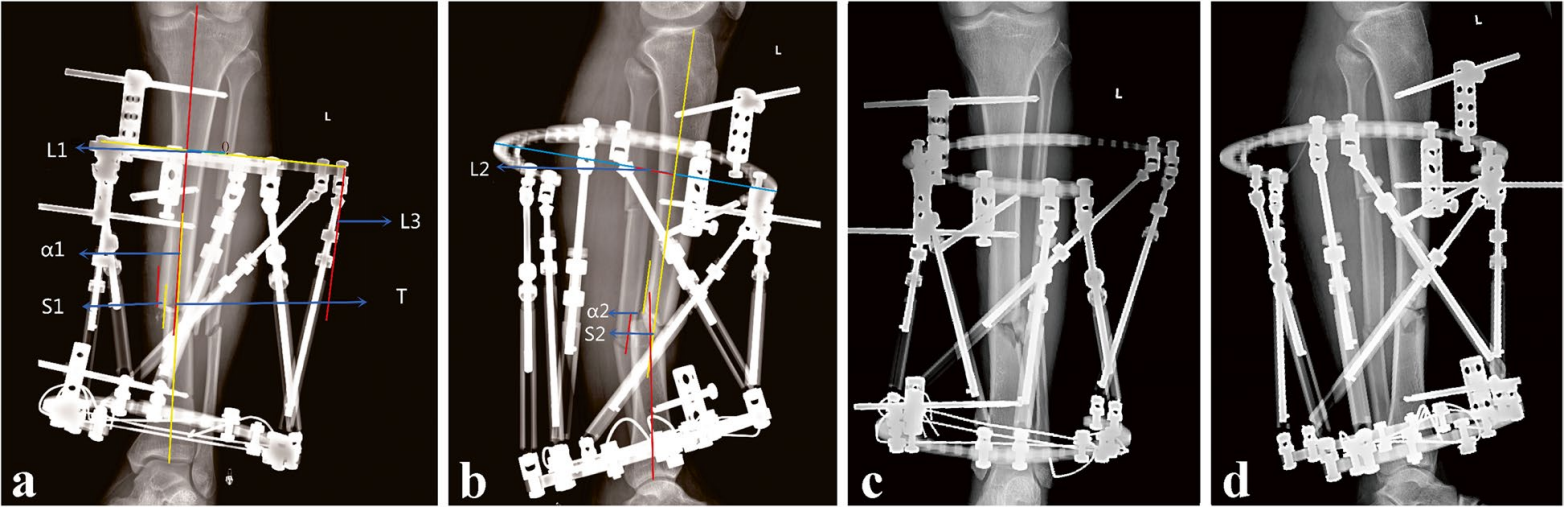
Radiographs showing patient with tibial fracture treated with TSF using traditional radiographic measurement method. Male,33 years old, left side. a Measuring deformity parameter in AP view. b Measuring deformity parameters in lateral view. c Immediate AP view after reduction. d Immediate lateral view after reduction
